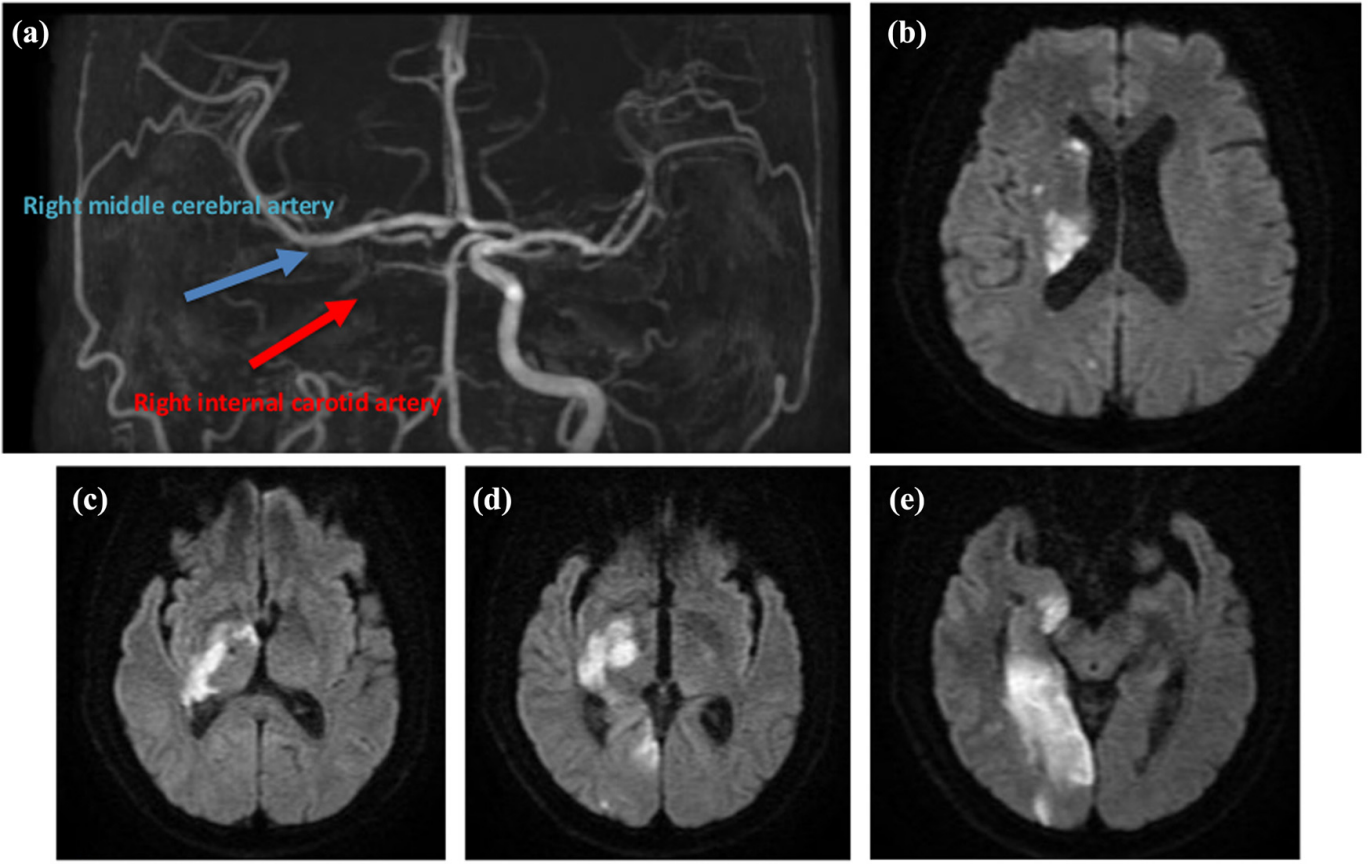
Initial neuroimaging findings. (a) Time-of-flight (TOF) MRA demonstrates occlusion of the right ICA and the right MCA, corresponding to the infarct distribution observed on MRI. (b–e) Diffusion-weighted brain MRI reveals acute infarcts involving the posterior limb of the right internal capsule, caudate head, hippocampus, and occipital lobe. Additional embolic infarcts are noted in the right cerebral hemisphere, along with two cerebral microbleeds. Blue arrow: The right MCA. Red arrow: The left ICA.
